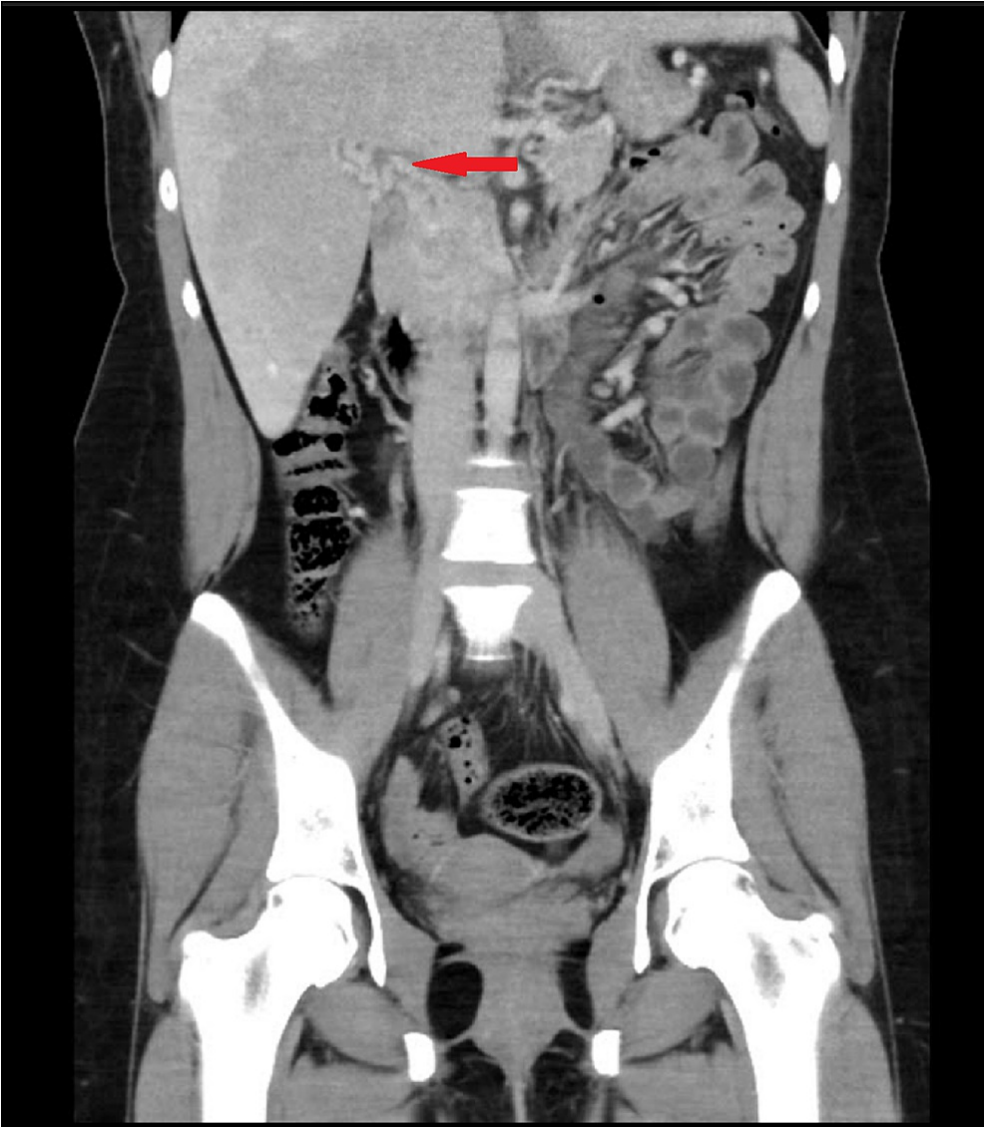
Progress CT scan CT Imaging six months after diagnosis showing persistent PVT with cavernous transformation of portal vein
